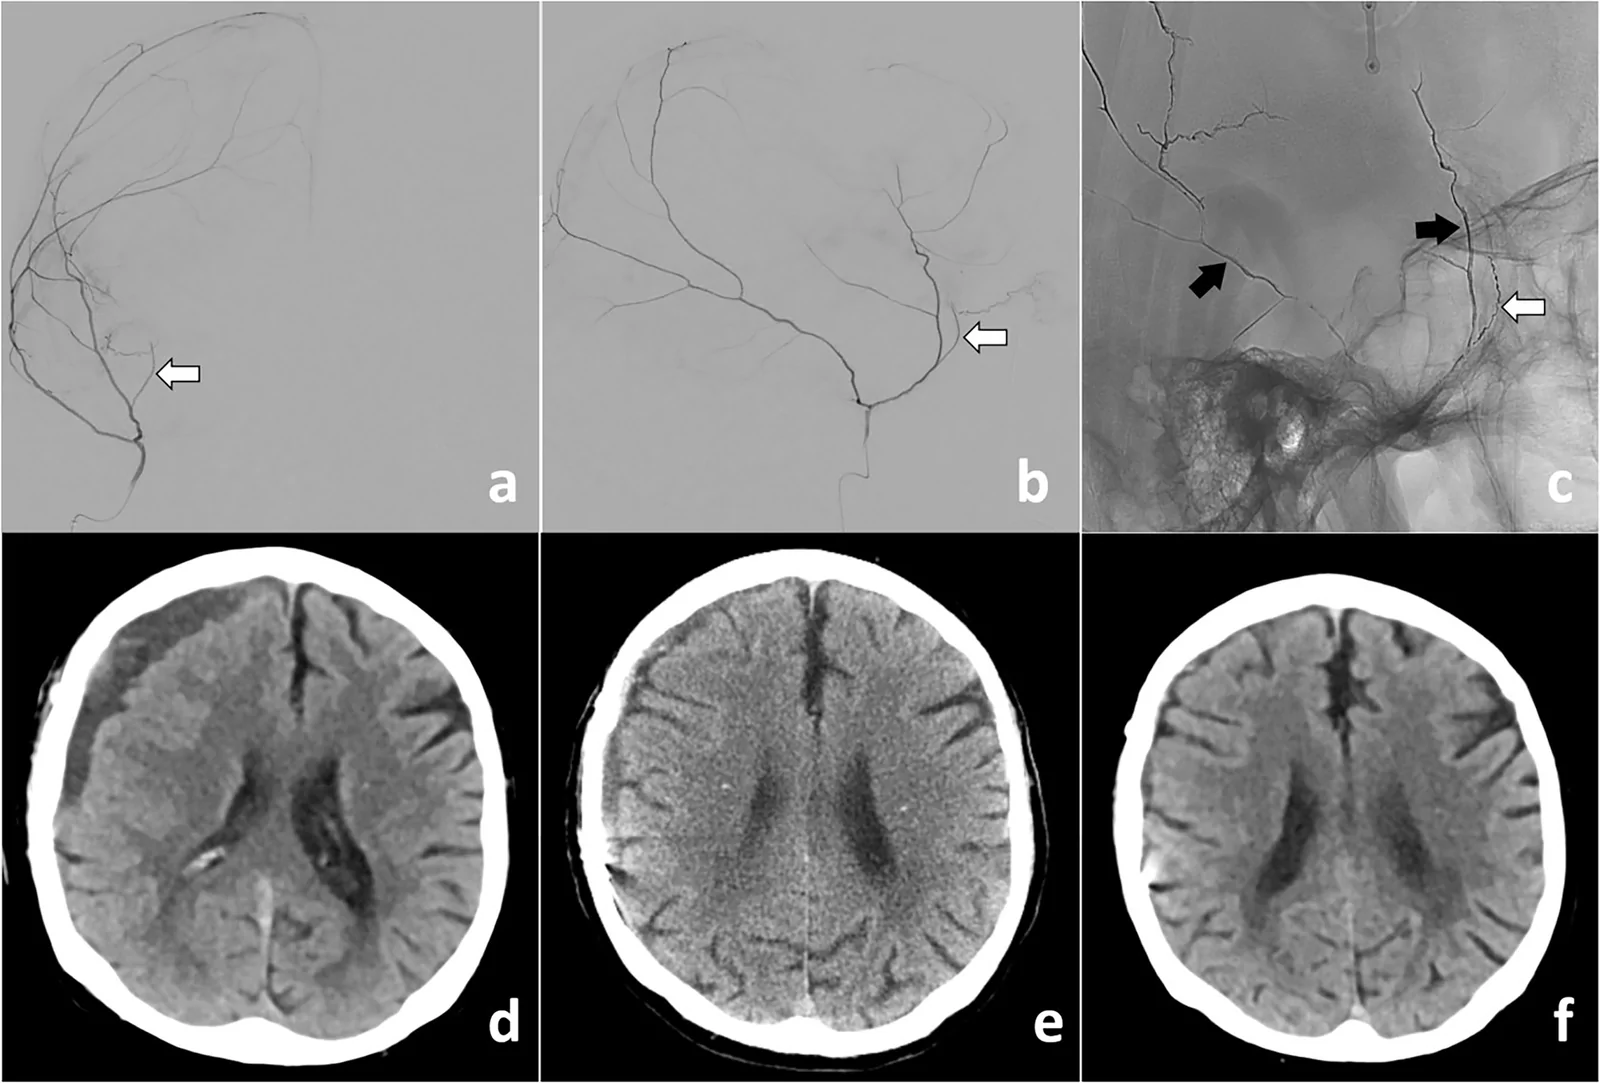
Middle meningeal artery embolization and follow-up in an 88-year-old male with recurrent chronic subdural hematoma. The patient underwent MMA embolization for a right-sided cSDH recurrence after initial surgical evacuation. a, b Superselective digital subtraction angiography (DSA) in frontal and lateral projections shows enlargement of the right MMA and its sphenoidal orbital branch (white arrow). c To prevent orbital complications, the orbital branch was coil-embolized (white arrow), followed by embolization of the frontal and parietal branches with liquid embolic agent (Onyx, black arrow). d–f Axial CT images demonstrate the hematoma volume post-procedure (d), showing partial resolution at 3-month follow-up (e), and complete resolution at 8-month follow-up (f)
